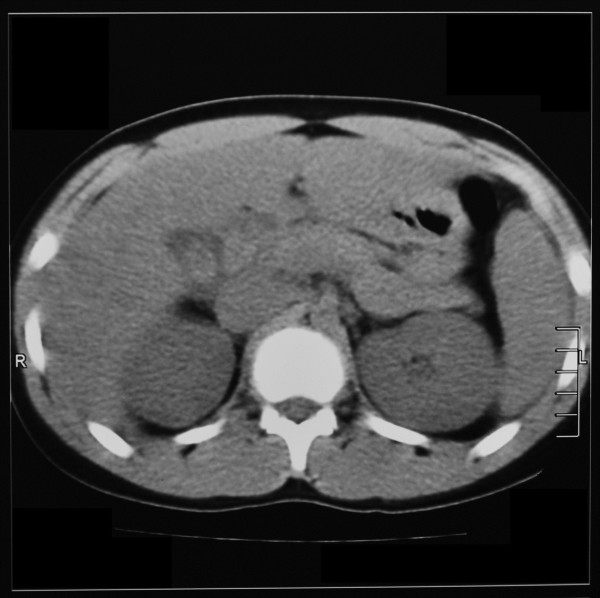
Non-contrast CT Abdomen of the patient demonstrating prominent edematous pancreas, pericholicysitc fluid along with hepatomegaly.
